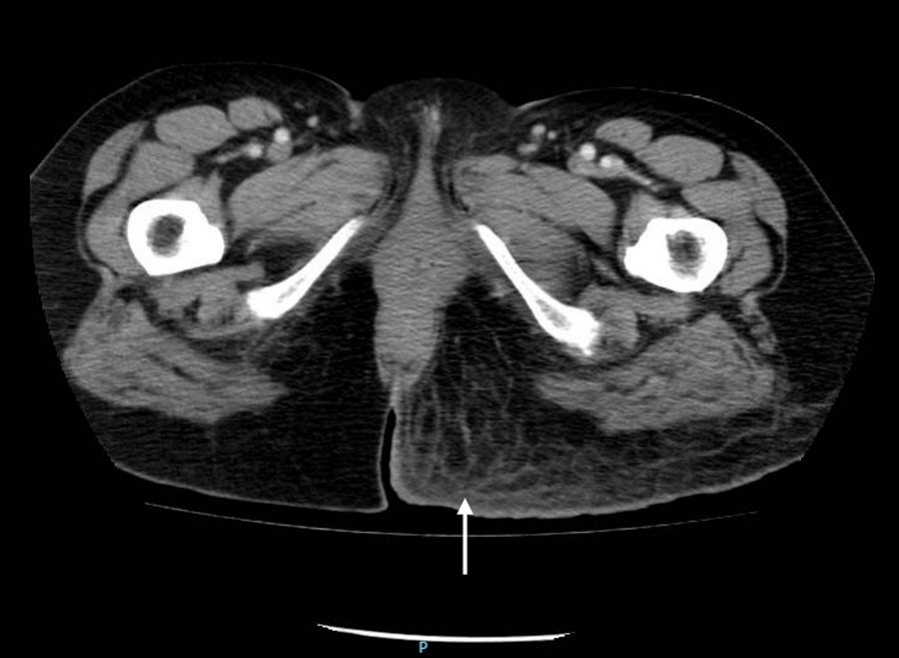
Contrast CT scan of the lower limbs on day 2 after surgical debridement. Axial tomogram at the level of the proximal thigh showing abnormal enhancement of the subcutaneous and fascial layer of the left gluteal region (arrow)
